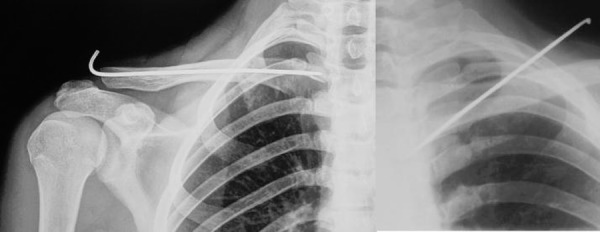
Xray shows a K-wire fixation on middle third clavicle fracture and the migration of K-wire.
